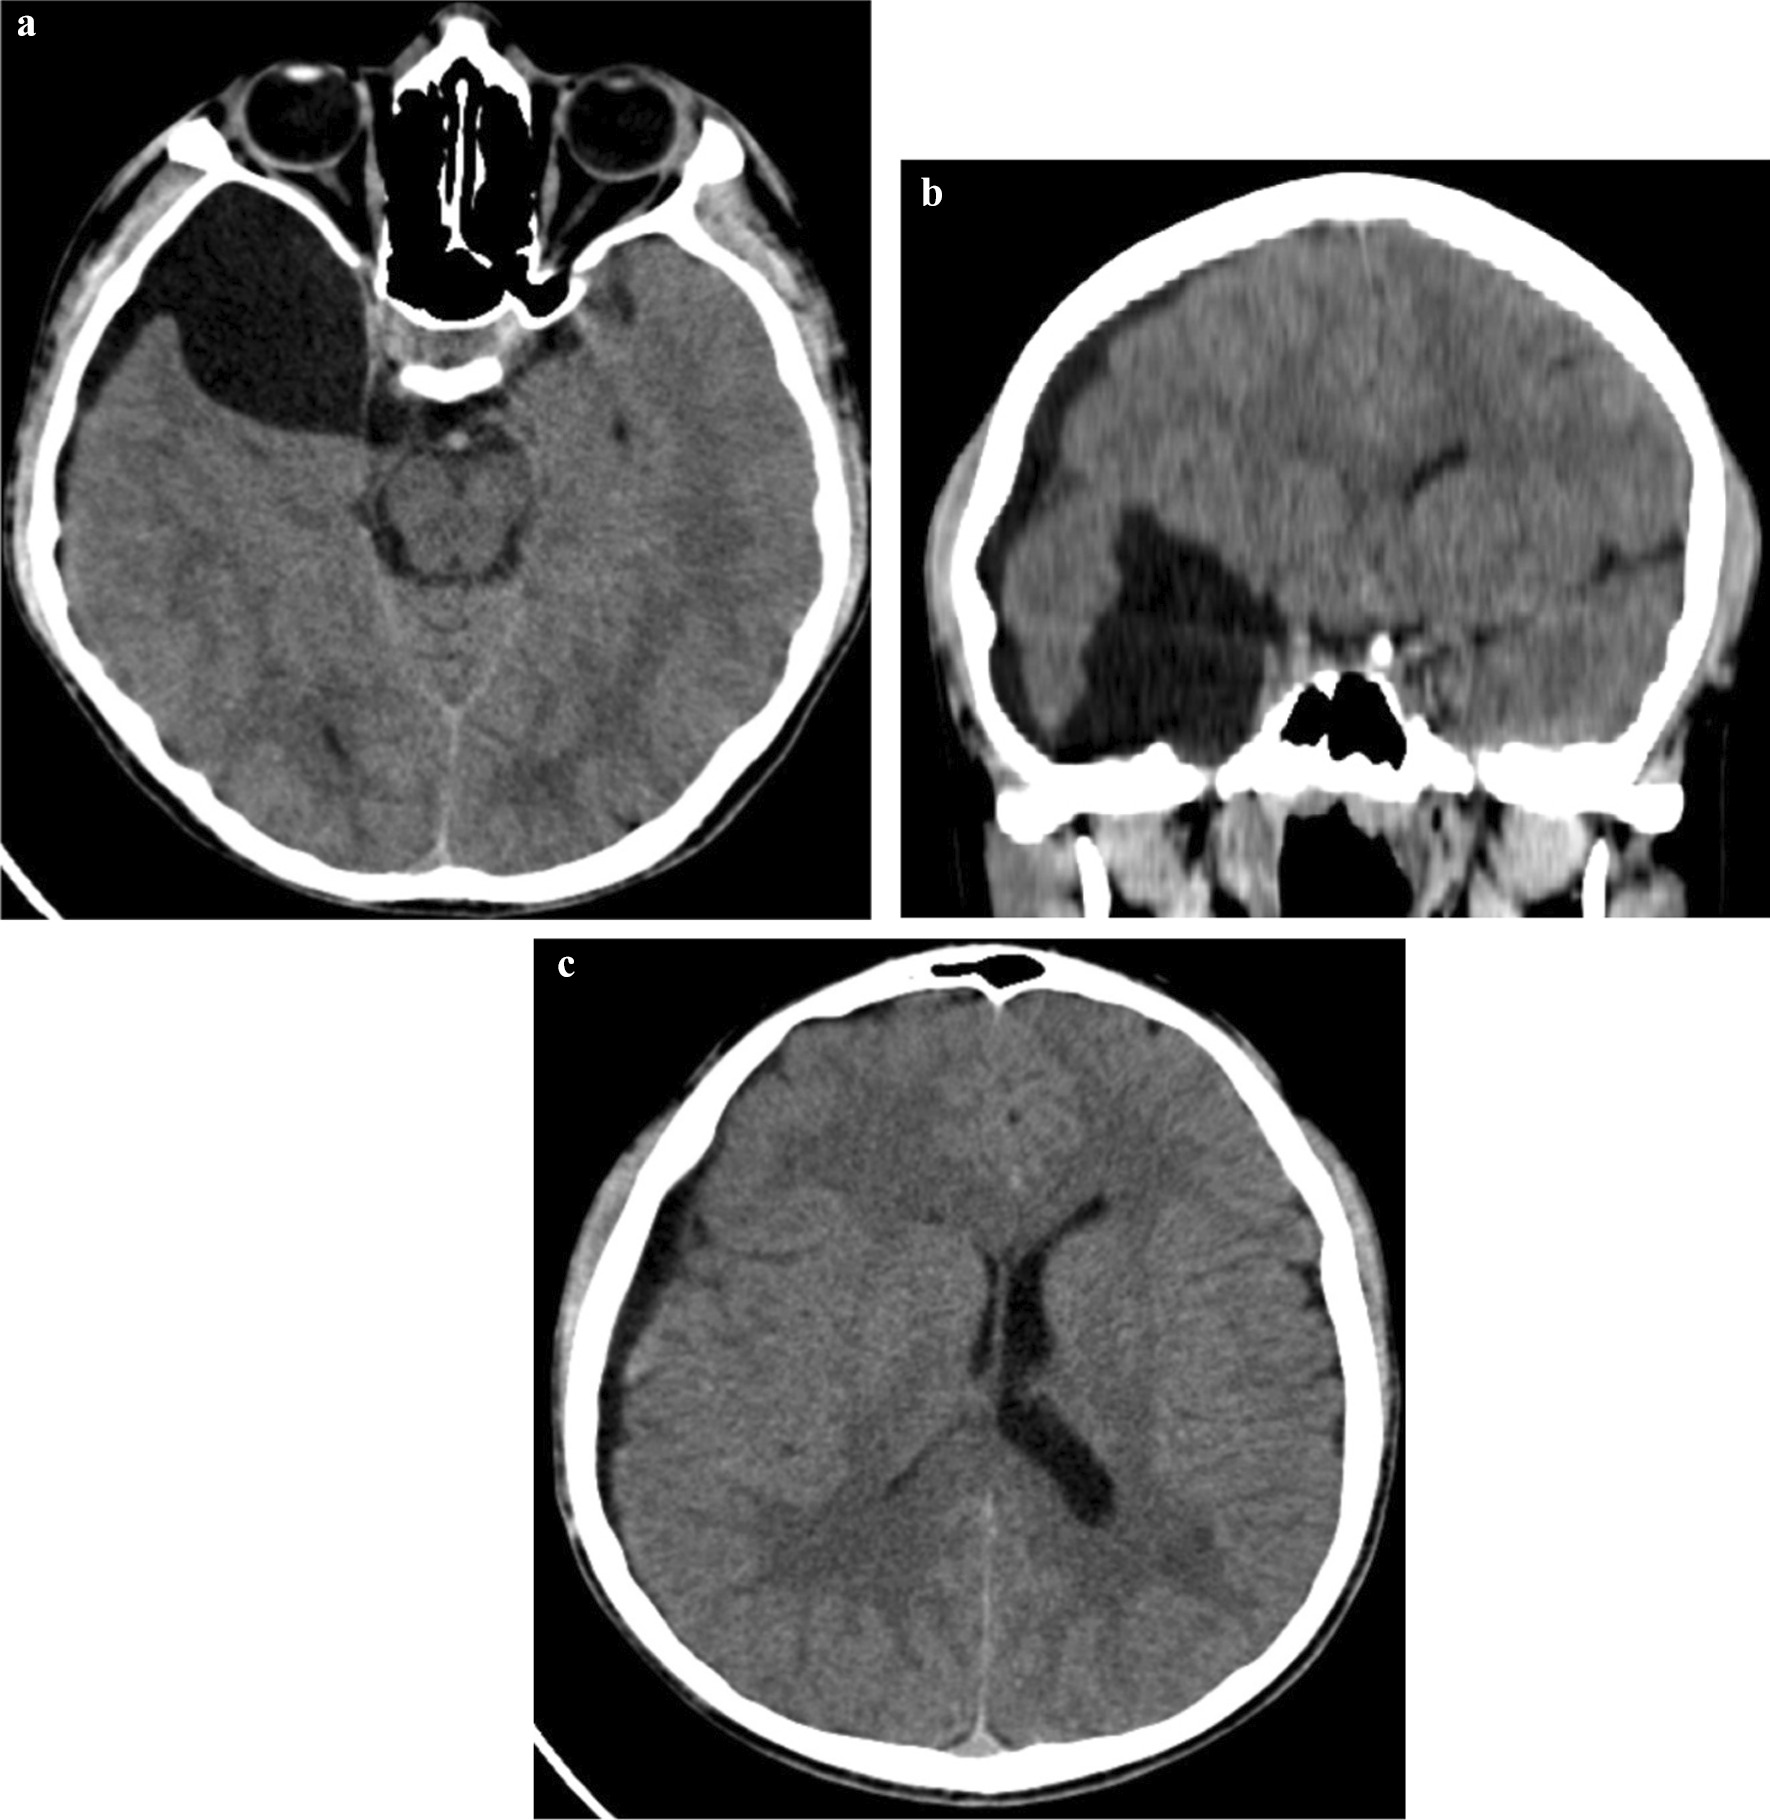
a Axial CT section through the temporal lobes: CSF intensity lesion in right middle cranial fossa anterior to left temporal lobe with mass effect. There is communication with subdural fluid in the vicinity of the temporal lobe. b Coronal CT section through the temporal lobes: CSF intensity lesion in right middle cranial fossa with atrophic changes of right temporal lobe. There is subdural fluid extending along the right frontotemporal lobes with resultant mass effect, midline shift, and effacement of right lateral ventricle. c Axial CT section at the level of lateral ventricles: subdural fluid along the right cerebral hemisphere, with mass effect, effacing the right lateral ventricle, mild midline shift, and prominent left lateral ventricle
